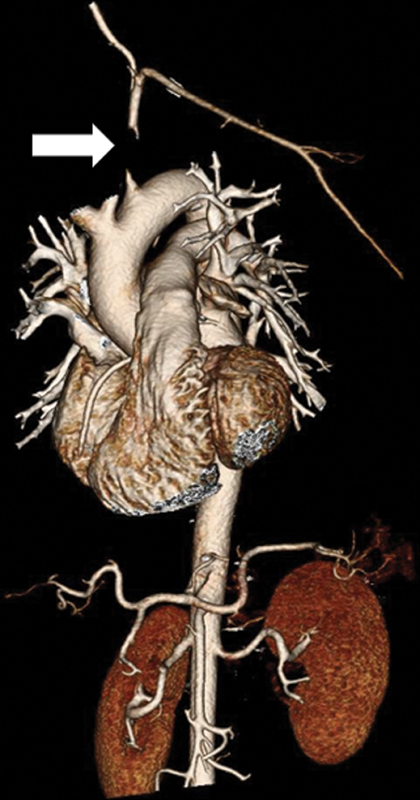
Computed tomography angiography three-dimensional reconstruction of the aorta and the left subclavian artery occlusion (white arrow).
